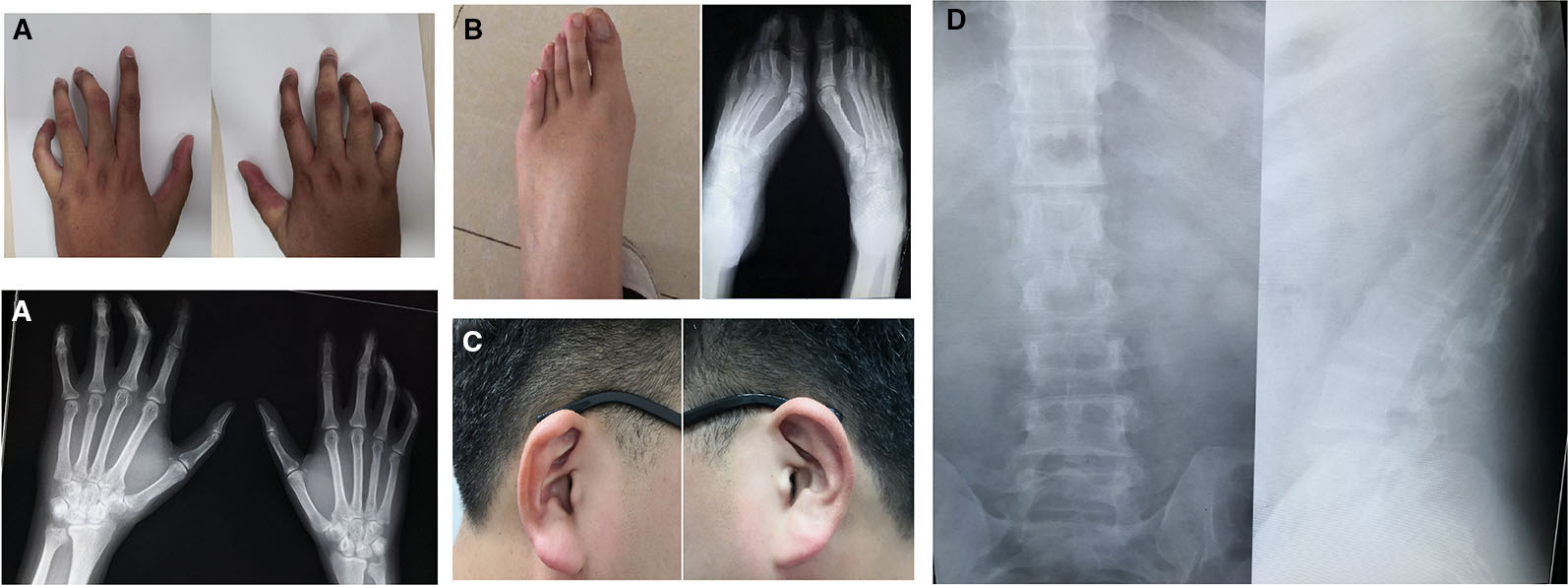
Clinical features of proband with congenital contractural arachnodactyly (CCA). (A) Photographs and x-ray plain film of fingers. (B) Photographs and x-ray plain film of toes. (C) Photographs of ears (after intervention). (D) Chest x-ray plain film.
